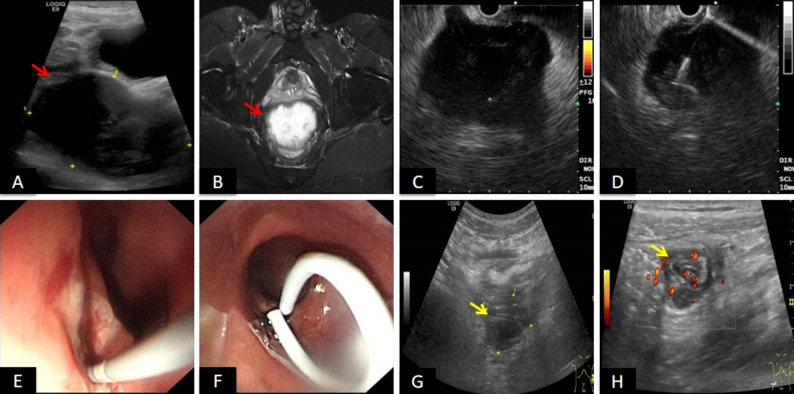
EUS-guided drainage for lager pelvic abscesses. A Intestinal ultrasound shows pelvic abscess (red arrow for abscess). B Transverse pelvic MRI shows pelvic abscess (red arrow for abscess). C EUS shows pelvic abscess and no blood vessels in the puncture approach. D Puncture needle is inserted into pelvic abscess under the guidance of EUS. E Fistulous tract is created by a cystotome. F Double-pigtail plastic stents are deployed through the fistulous tract into the abscess cavity. G Intestinal ultrasound shows significant reduction in pelvic abscess size one week later (yellow arrow for post-procedure resolution). H Intestinal ultrasound shows that pelvic abscess had almost disappeared five weeks later (yellow arrow for post-procedure resolution)
